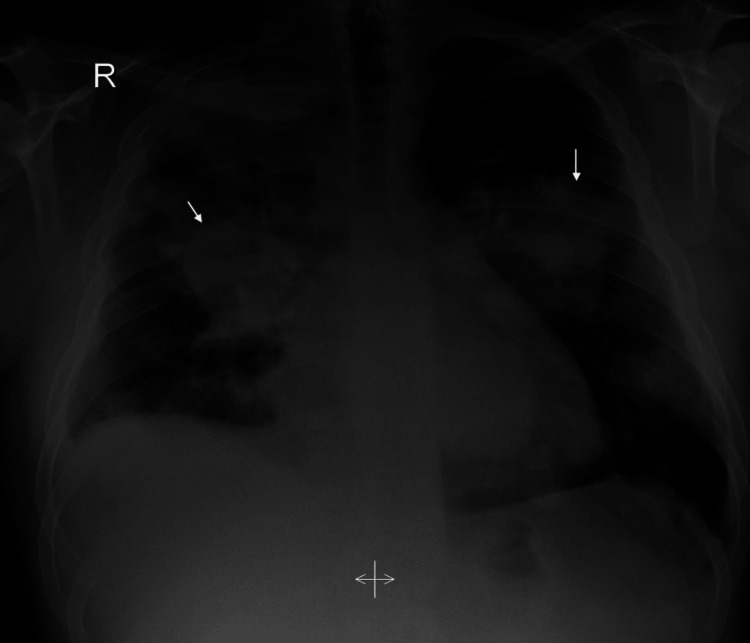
Chest X-ray: bilateral patchy dense opacities with marked hilar enlargement and a blunted right costophrenic angle
